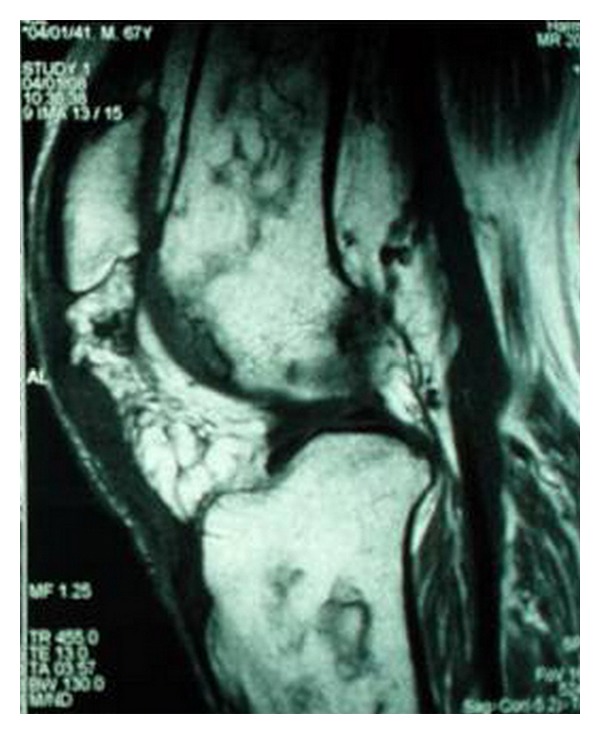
MRI of the left knee reveals complete rupture of the patellar tendon and patella alta.
